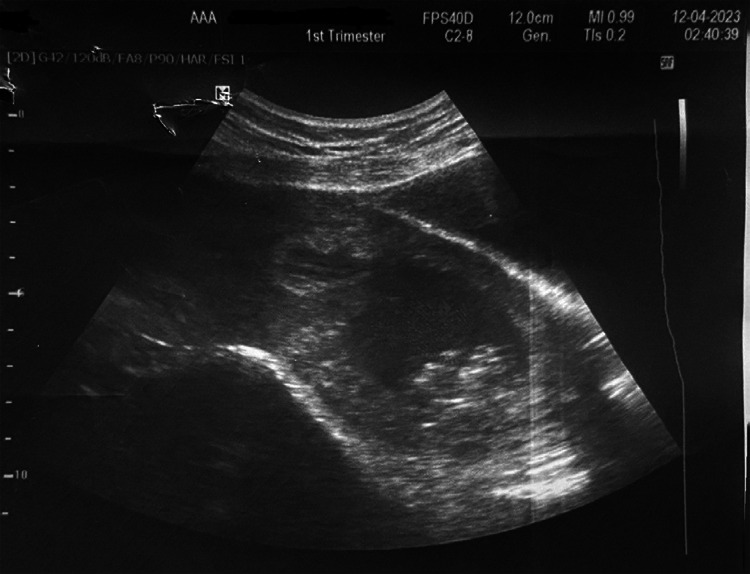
Ultra sonogram showing endometrial thickness.
